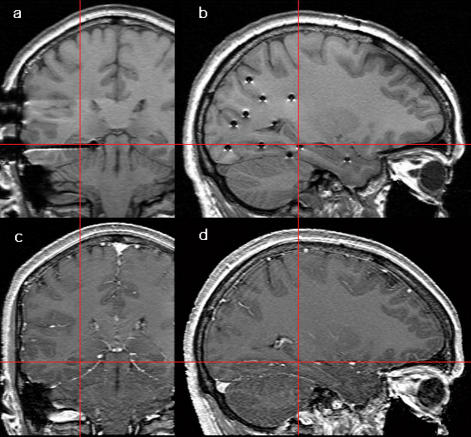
Magnetic resonance imaging (MRI) scan of intracranial electrodes implanted into the medial temporal lobe (a and b panels, coronal and sagittal views, respectively). Red line cross indicates the location of the two electrode contacts within the hippocampus. C and d panels show the MRI scan of the same locations before the electrode implant.
